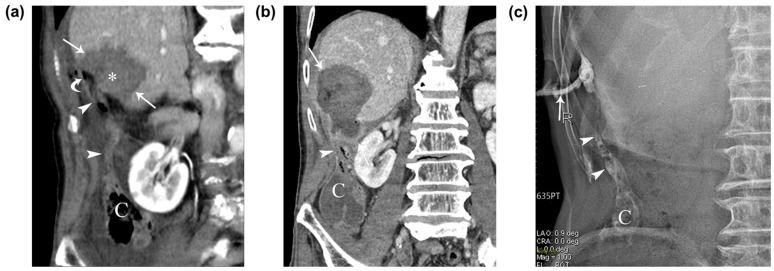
An immediate post-RFA contrast-enhanced CT scan demonstrated a low-attenuating ablated area that covered the tumor and the air-filled appendix, which extended superiorly from the cecum to the perihepatic space near the ablated zone of segment 5 of the liver, with a thickened appendiceal tip (Figure 2a). The patient was closely monitored and treated with intravenous antibiotics. On the fifth day after RFA, the patient’s laboratory results showed elevated aspartate aminotransferase and alanine aminotransferase levels, as well as an elevated high-sensitive C-reactive protein level. The patient presented with intermittent right upper abdominal pain and chills after the RFA, and a follow-up CT scan performed 7 days later revealed the formation of an abscess in the ablated zone and adjacent perihepatic space, although communication between the appendix and the ablated zone was not clear on the CT scan. A 10-French pigtail percutaneous drainage catheter was inserted into the hepatic abscess between the ablated zone and the perihepatic appendix (Figure 2b), and fluoroscopic imaging showed a fistulous tract between the ablated zone and appendix (Figure 2c). Abscess formation after percutaneous ultrasound-guided radiofrequency ablation for a 1.2 cm subcapsular hepatocellular carcinoma in segment 5 of the liver. (a) Immediate follow-up contrast-enhanced coronal CT image after ultrasound-guided radiofrequency ablation depicts a low-attenuating ablated area (arrows) which sufficiently covers the tumor (asterisk) and an air-filled perihepatic appendix (arrowheads) with a thickened tip from cecum (C). Note the small air density (curved arrow) between the ablated area and the appendiceal tip. (b) Follow-up contrast-enhanced coronal CT image taken 7 days after the RFA depicts abscess formation in the ablated zone and adjacent perihepatic space (arrow) and a thickened perihepatic appendix (arrowheads). (c) A 10-French pigtail percutaneous drainage catheter (arrow) was inserted into the sinus between the ablated area and the appendiceal tip. The fluoroscopic image depicts the contrast-filled appendix (arrowheads) with mottled air density and cecum (C). After 1 month, the patient’s clinical symptoms and laboratory findings subsided and improved, and a follow-up tubogram showed an improving abscess with a remaining fistula. Surgical treatment was initially considered, and the potential difficulties due to postoperative adhesion and potential postoperative complications were explained to the patient. However, the patient opted against this approach, leading us to decide on a conservative treatment. The patient was discharged following the removal of the percutaneous drainage catheter.
